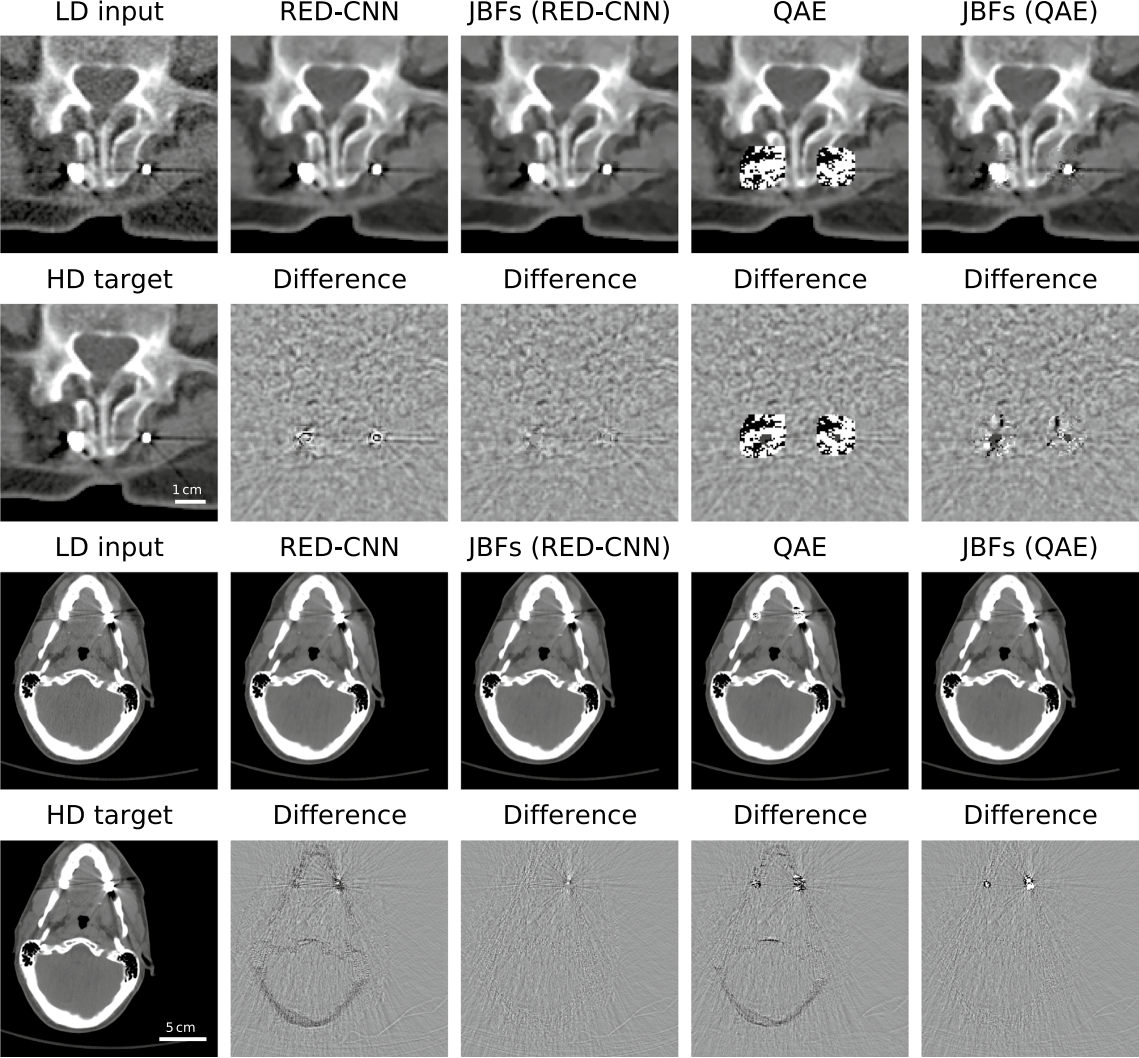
Qualitative denoising results on the ROI highlighted in Fig. 3 and on a head CT slice. Difference images are calculated between model prediction and high-dose (HD) target and are shown in the window $$[-50, 50]\,\text{HU}$$ for abdomen data and $$[-100, 100]\,\text{HU}$$ for head data. The reconstruction window is $$[-150, 500]\,\text{HU}$$. Our hybrid models visually outperform the respective vanilla deep models.
